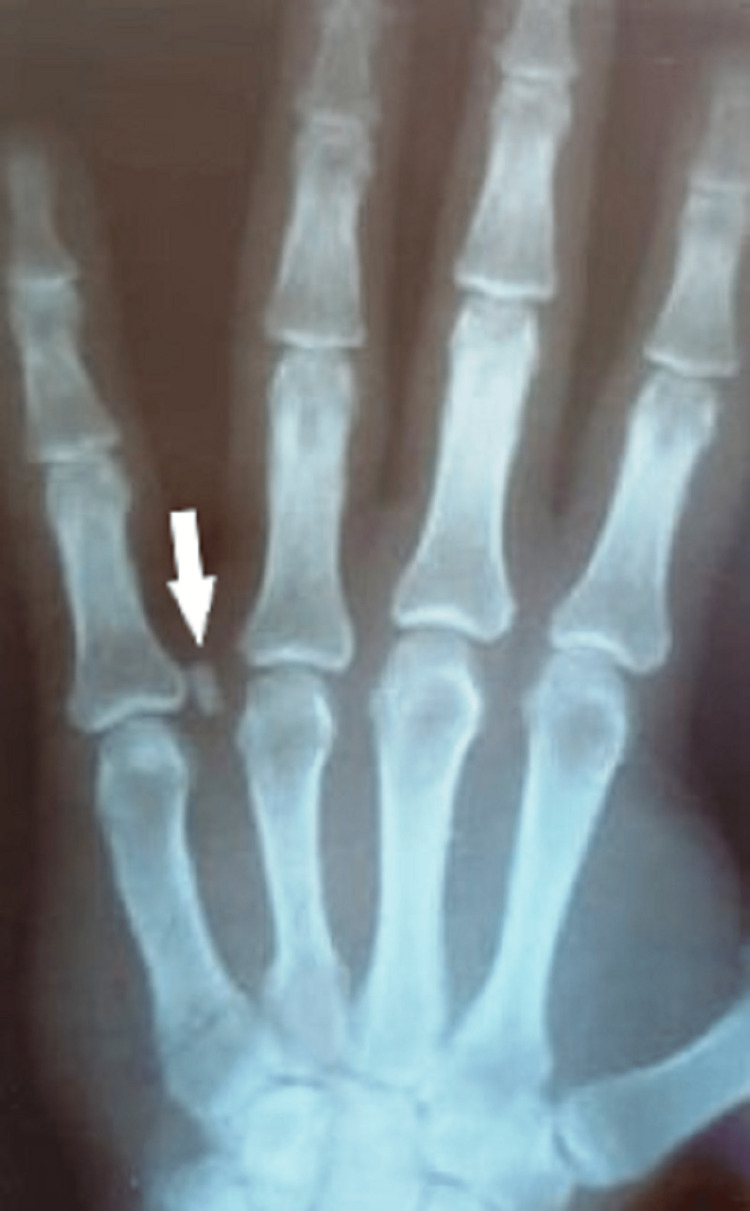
Left-hand X-ray showing well-limited soft tissue centimetric calcification.
